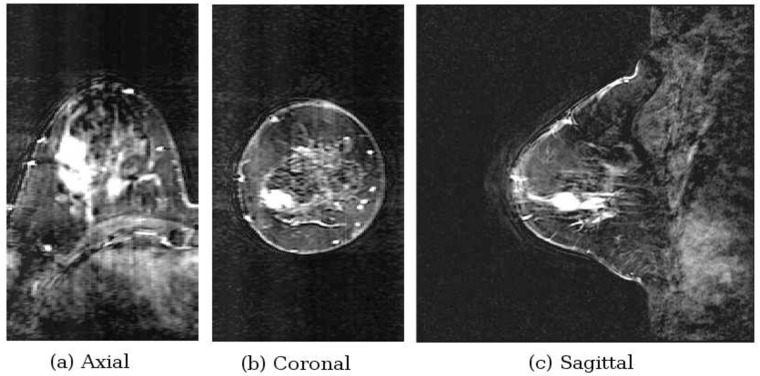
Contrast enhanced MR images for Patient 130920. This image shows the presence of a tumor (bright white region).
